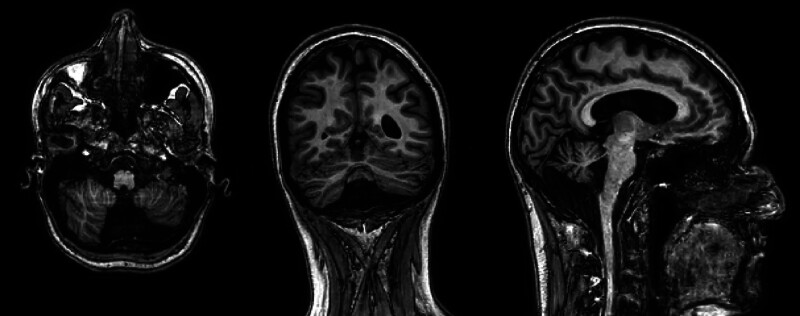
Structural MRI of the case presentation.
